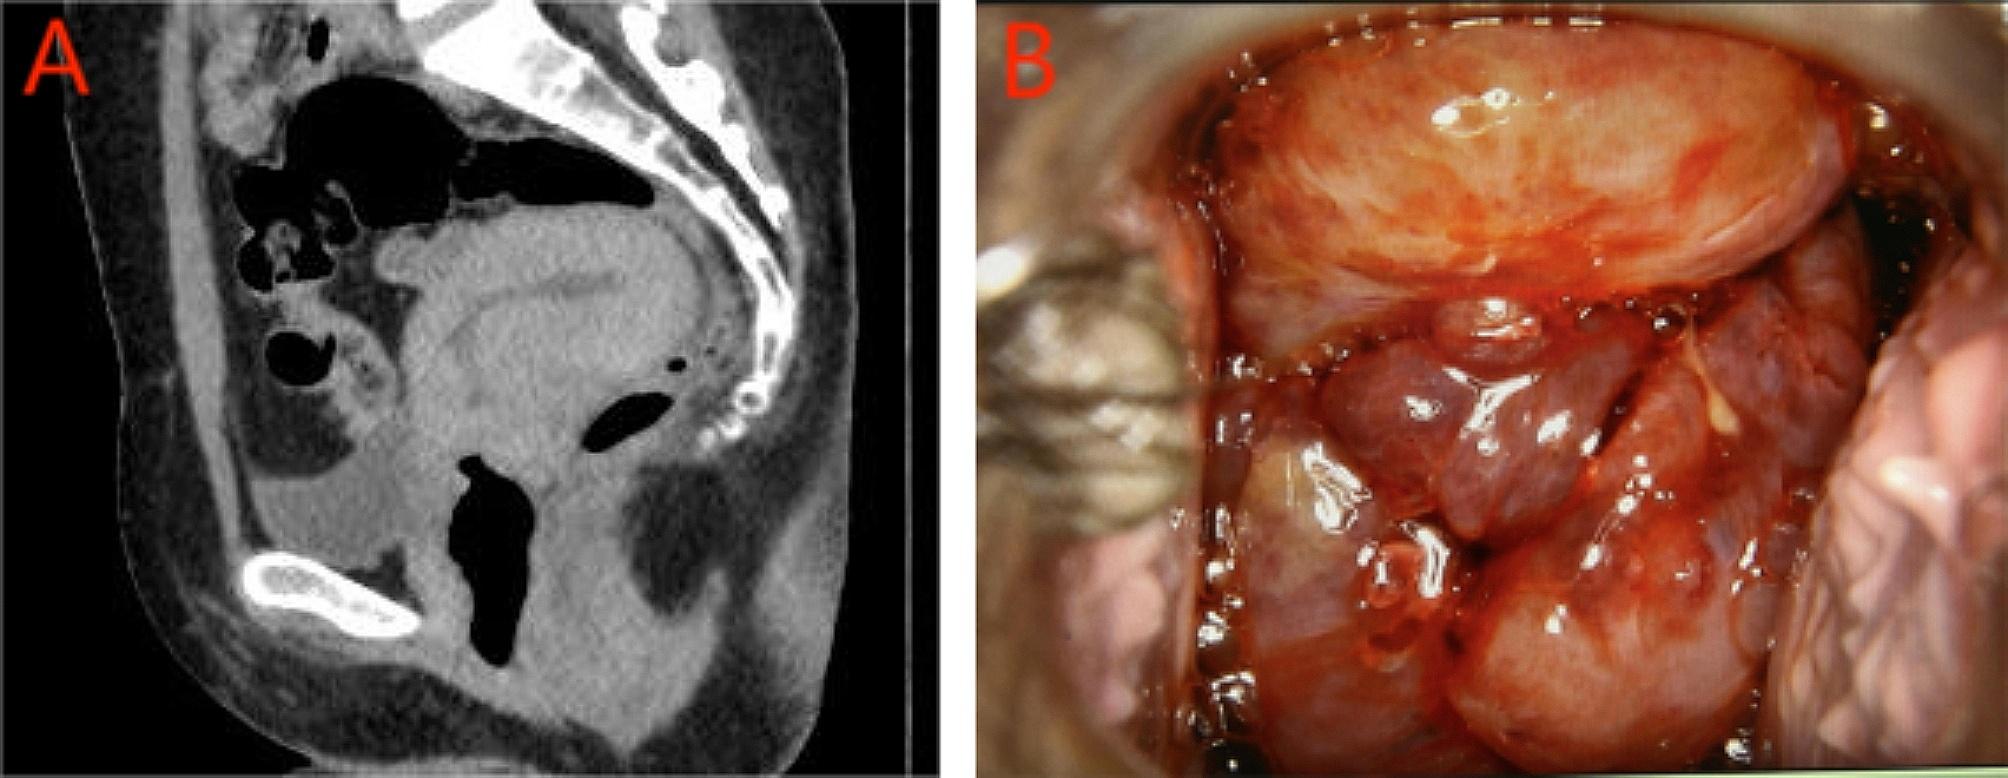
CT revealing an abnormal soft tissue nodule shadow in the cervical canal and an outer cervical opening with a long diameter of approximately 3 cm and uneven enhancement after enhancement (A). A cervical lobated neoplasm (4 × 4 cm) was observed via colposcopy. The neoplasm was grape-like in appearance (B)
